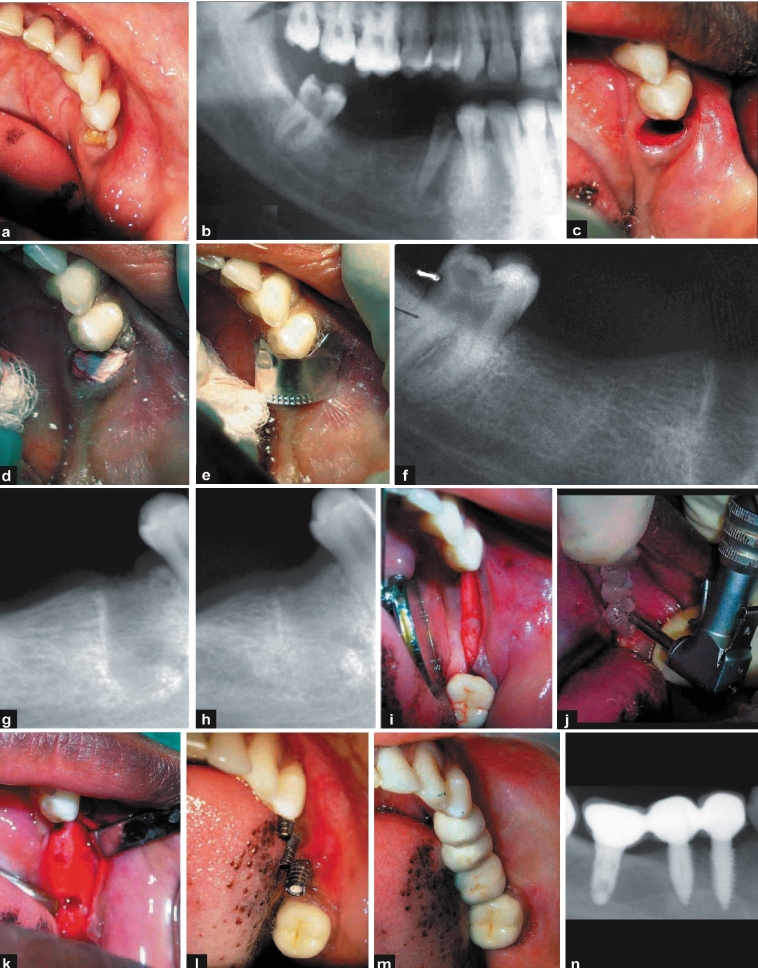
a, b) Pre-operative photograph and radiograph; c-f) operative photographs and radiograph during socket preservation; g, h) 1-month and three-month radiograph post socket preservation; i-k) implant placement photographs; l-n) post-operative photographs and radiograph
